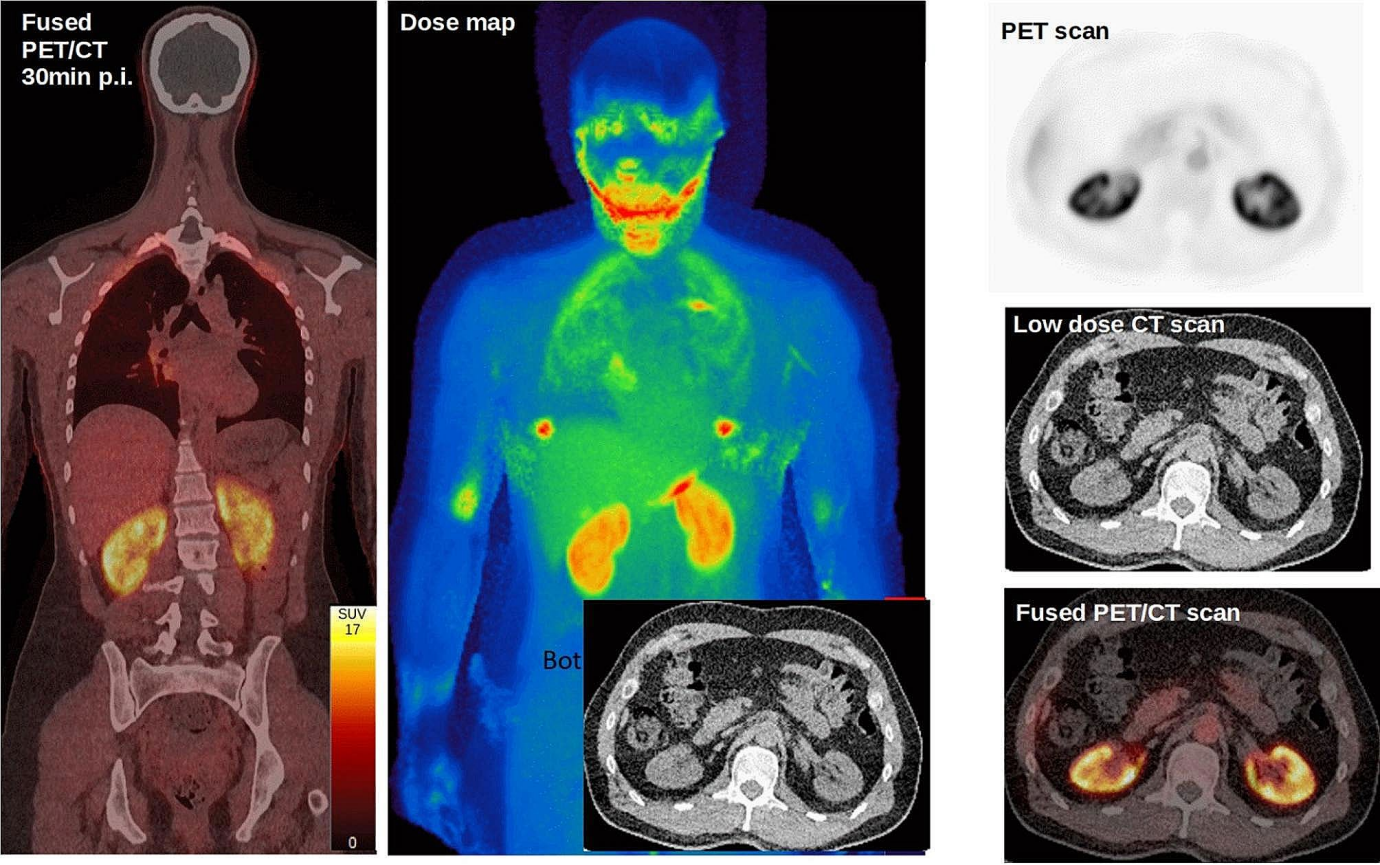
Biodistribution of [^18^F]Me4FDG. Left: Fused PET/CT image of the last scan at 270 min showing the biodistribution of [^18^F]Me4FDG with the highest concentration measured in the renal cortices. Middle: Dose map in units of mSv of the same individual. Right: Transaxial view of the kidneys in the PET images, the low dose CT images as well as the fused scans
